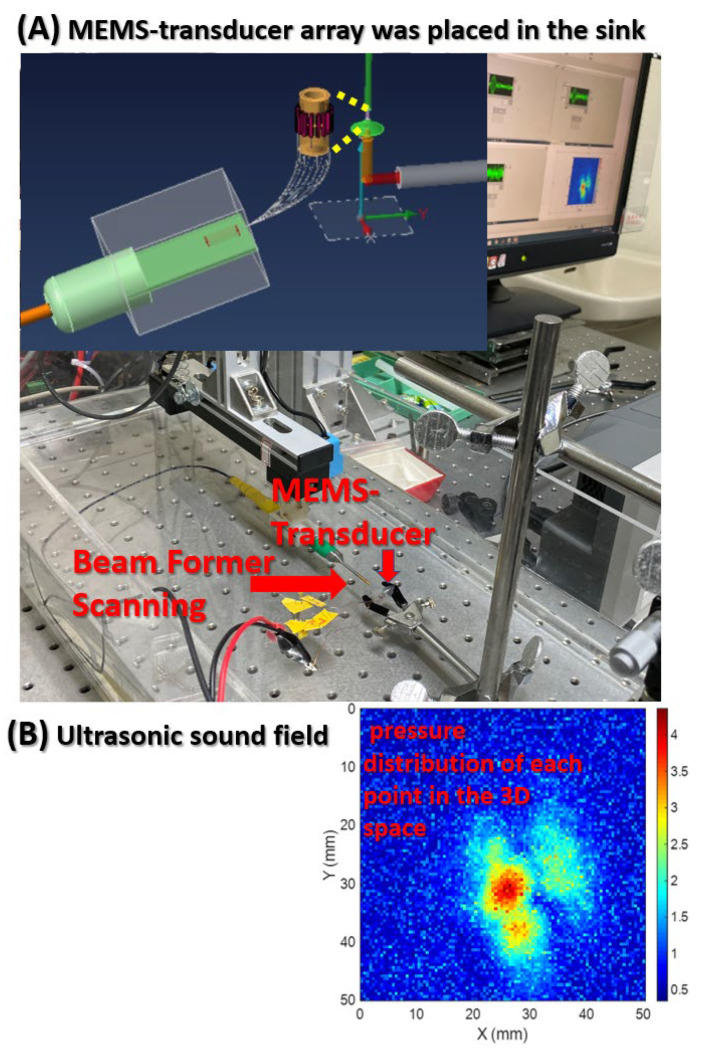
Beamforming was performed to scan the ultrasonic resonance energy of the MEMS-transducer. (A) The 16-channel circular MEMS-transducer array was placed in the sink for ultrasonic signal monitoring. (B) 10.00 Vpp signals were sent to Channel 5 at a frequency of 2.3759 MHz. Beamforming was performed to detect ultrasound signals.
